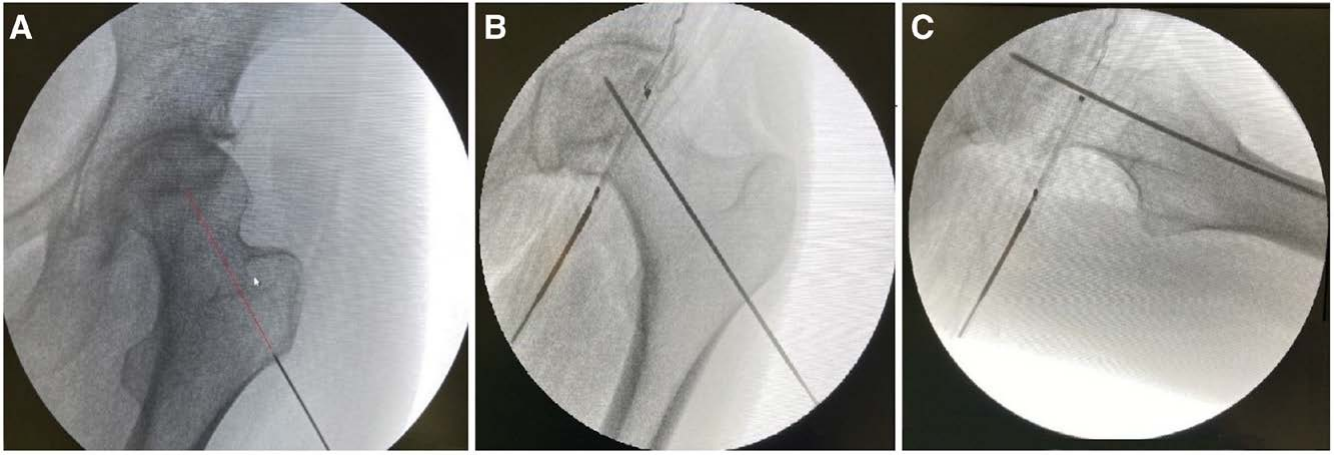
(A) Anteroposterior X-ray view of the avascular femoral lesion. (B) Anteroposterior view of guide K-wire application. (C) Lateral X-ray view of the K-wire application.
